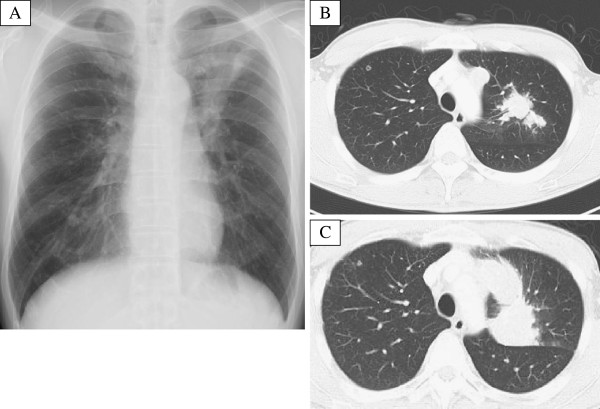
A. Chest radiography revealed a mass shadow in the left upper lung field. Figure 1B, 1C. Compared with the initial computed tomography (CT) of thorax (B) and the repeated CT at 30 days after erlotinib treatment (C).
